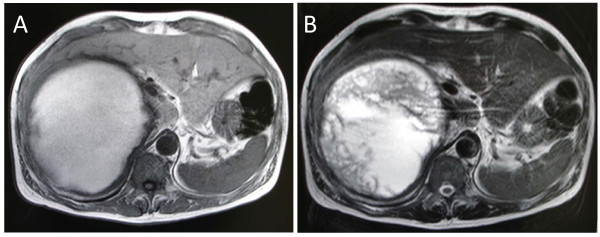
Abdominal magnetic resonance imaging revealed a well-defined high intensity mass which appeared homogeneous intense in the T 1-weighted image (A) but heterogeneously intense in the T 2-weighted image (B).
